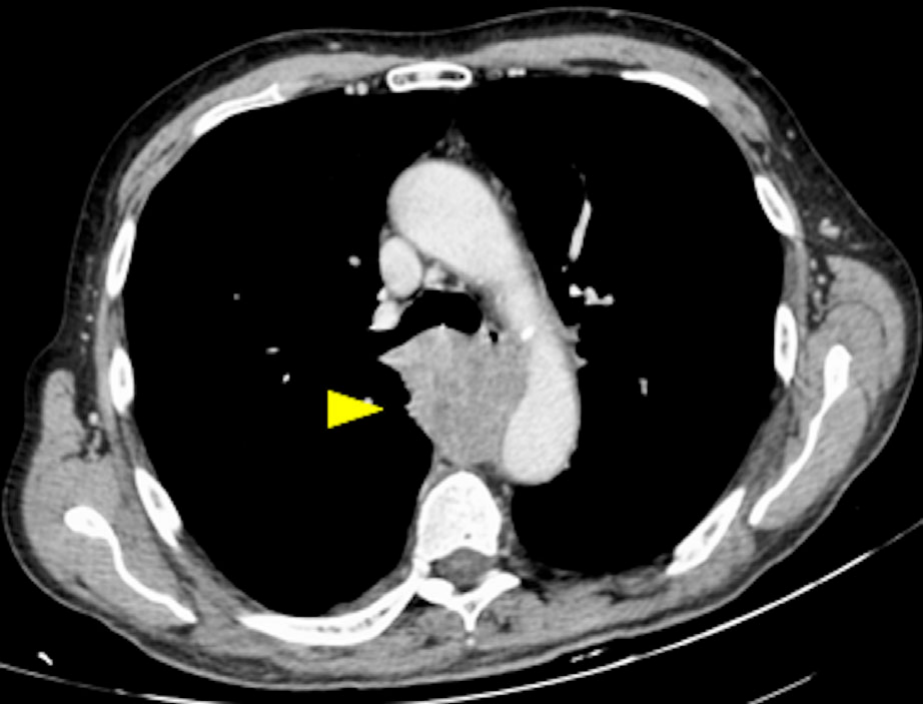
Chest computed tomography (CT) scan. Mediastinal window image shows a solid lesion in the posterior mediastinum (yellow arrow head).
